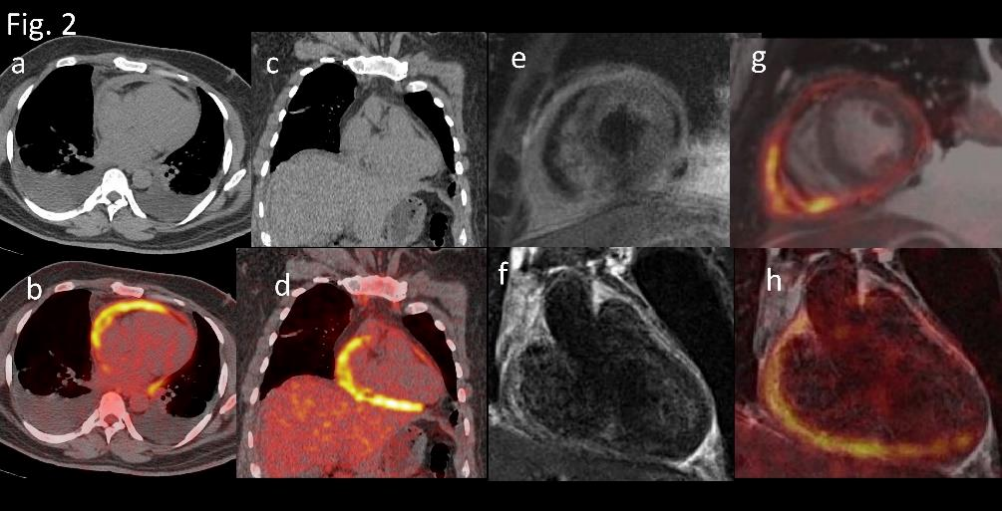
a,c,) Plain CT b,d,) PET CT images reveal hypermetabolic pericardial wall thickening and bilateral pleural effusion. e) Two chamber short and f) long axis T2TSE MRI and g,h) corresponding sequential fusion PET MRI reveal pericardial thickening appearing heterogeneously hyperintense on T2 WI corresponding to the hypermetabolic pericardial thickening on PET CT
